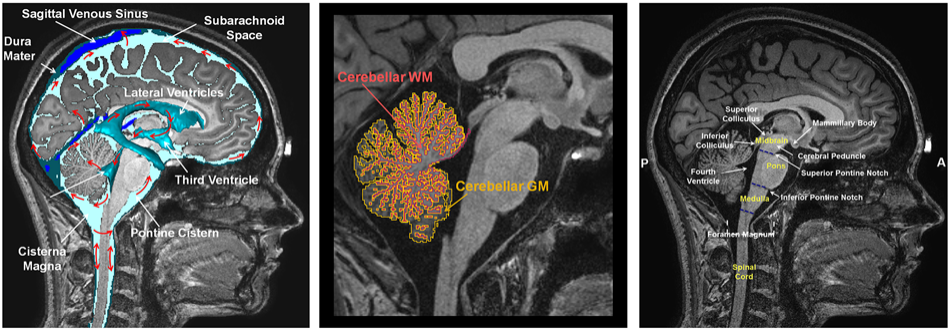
Segmentation of the brainstem, cerebellum, and CSF. Mid-coronal slice of the T1-weighted MRI (left) showing the CSF circulation (red arrows). Magnified view of the mid-coronal slice of the cerebellum (middle), showing the segmentation of the cerebellar GM (yellow) and WM (red). Mid-coronal slice of the brainstem and spinal cord (right), showing the landmarks used to subdivide the brainstem into its constituent substructures, i.e., the midbrain, pons, and medulla, and to separate the brainstem from the spinal cord.
